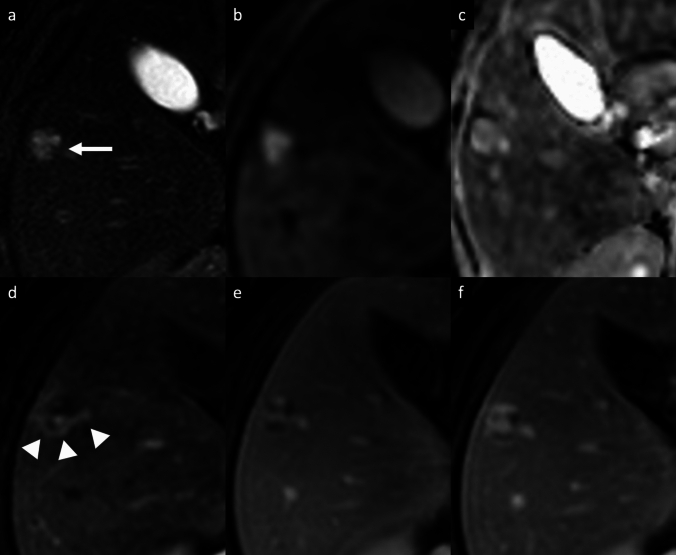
Cavernous hemangioma in a 67-year-old woman with hepatic steatosis. Magnetic resonance images show a lobulated focal liver lesion in the right hepatic lobe with high signal intensity on fat-suppressed T2-weighted image (arrow, a), high signal intensity on diffusion-weighted images (b) and high signal intensity on ADC map (c). On extracellular contrast agent-enhanced MRI, the lesion demonstrates peripheral discontinuous nodular enhancement on arterial phase (arrowheads, d), followed by progressive centripetal incomplete enhancement on portal venous (e) and delayed phases (f)
